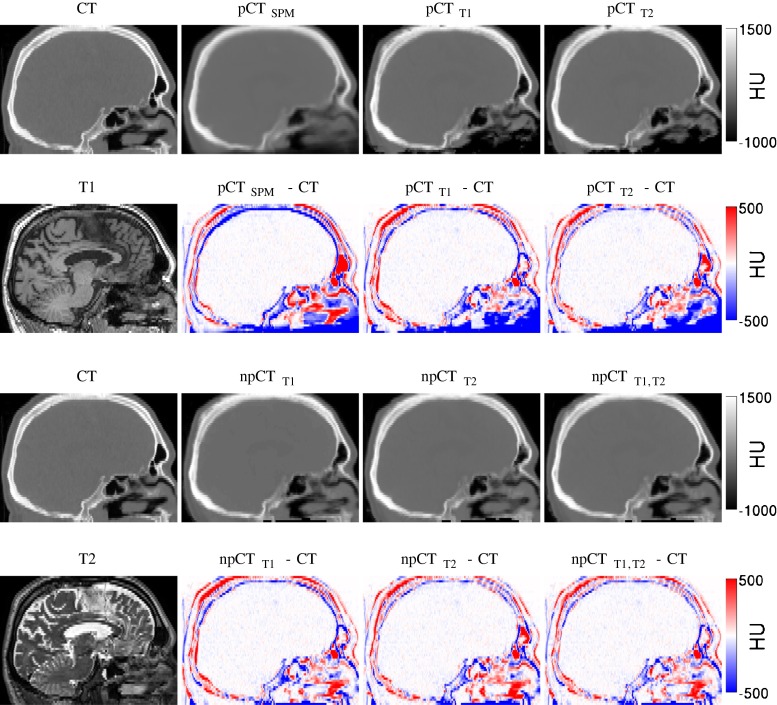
For a representative subject, the acquired CT, T1 and T2 images (left), and the pseudo CTs with the associated difference images
